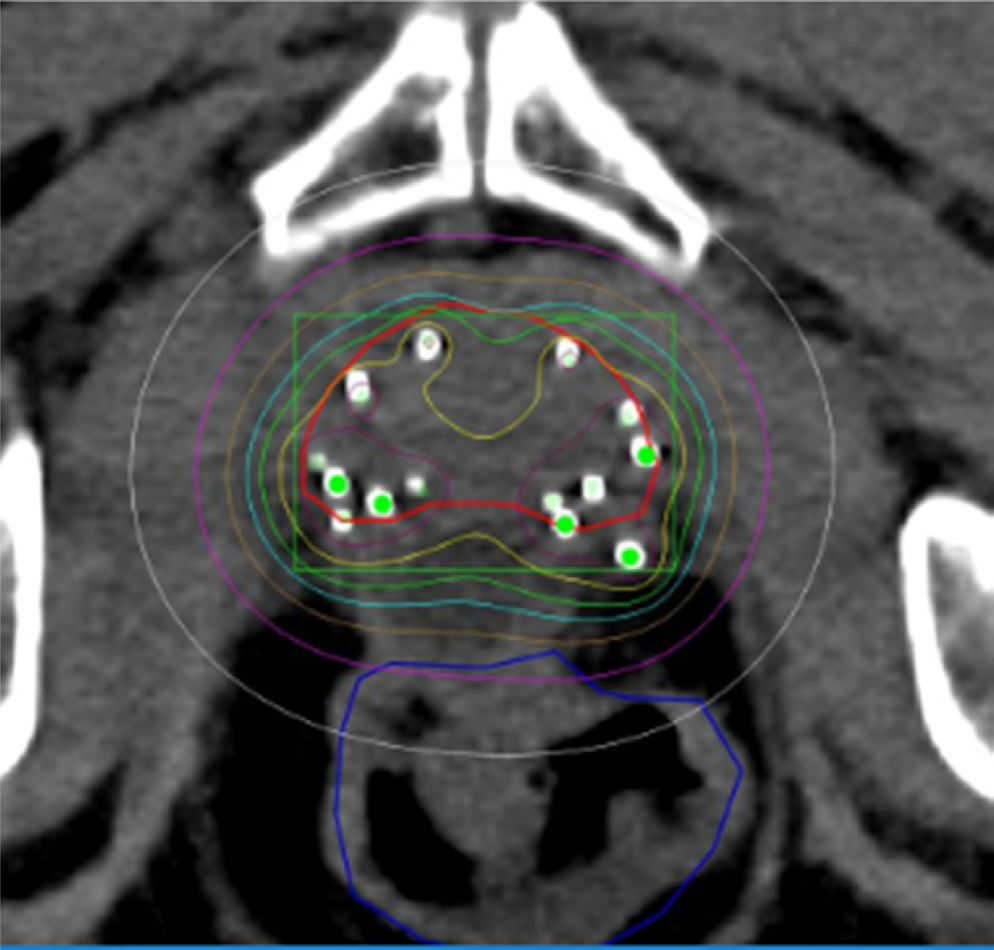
Isodose distribution in an axial CT plane after ^125^I brachytherapy implantation with a RBI. The prescribed dose to the prostate (red) was 145 Gy (green). The high-dose region >120% (yellow) and the low-dose region <40% (purple) with minimal overlap in the rectum (dark-blue). *Abbreviation*: RBI = Rectal Balloon Implant. (For interpretation of the references to color in this figure legend, the reader is referred to the web version of this article.)
